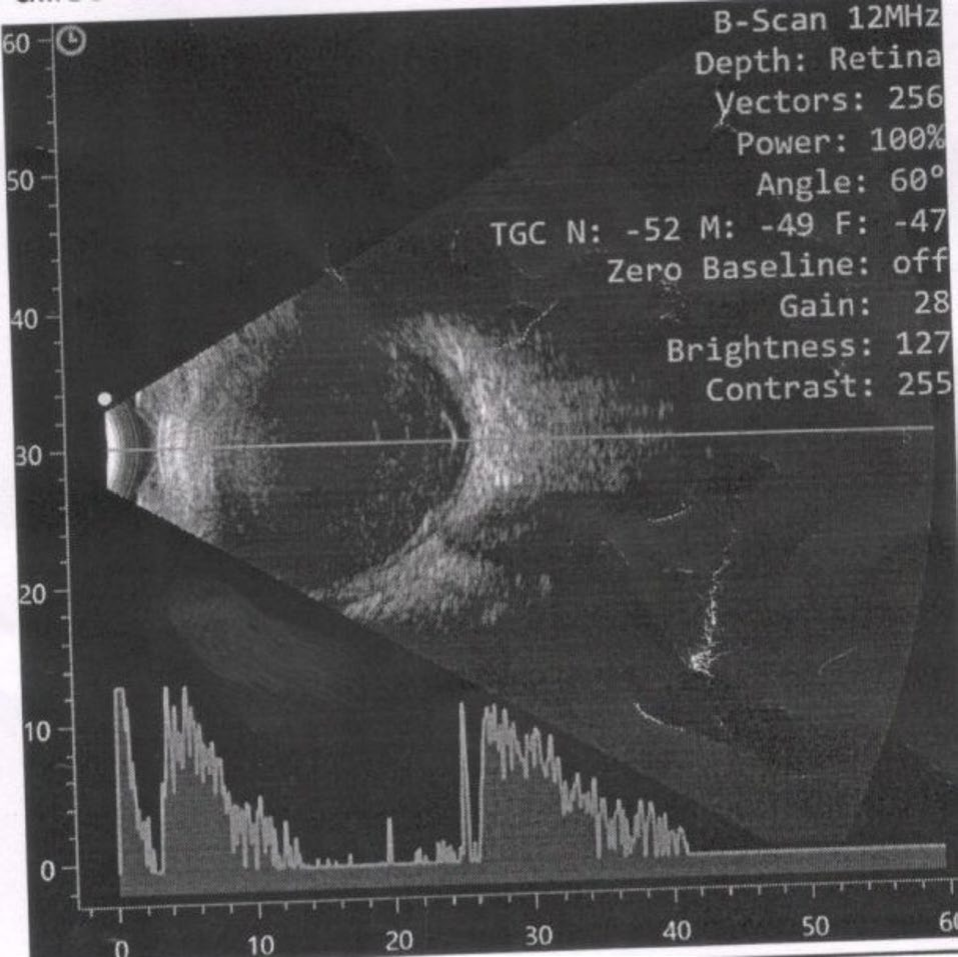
B scan ultrasonography at presentation: Shows a large number of low-to-medium reflective dots, clumps, and membranous echoes in the vitreous with choroidal thickening and an attached retina
